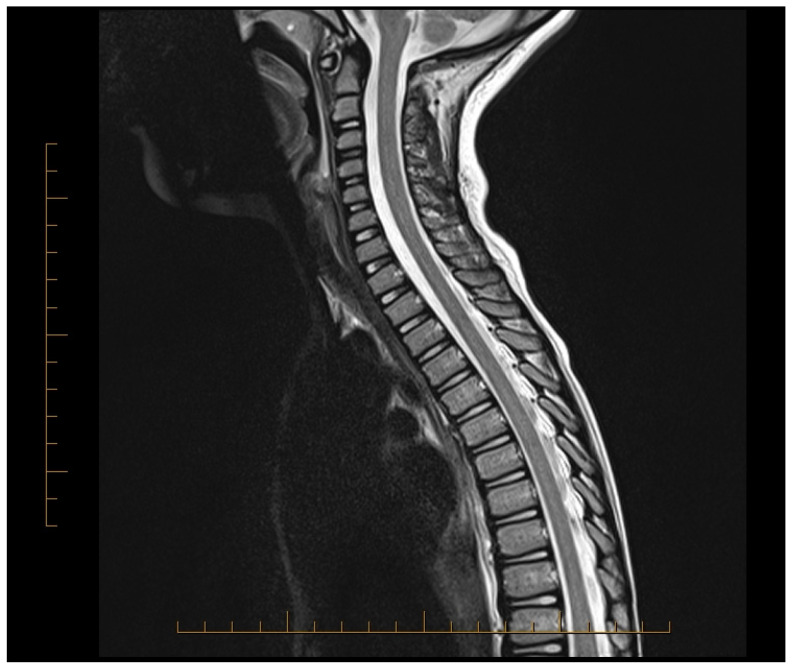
MRI of the spinal cord showing spinal cord lesions improved in appearance after treatment.
